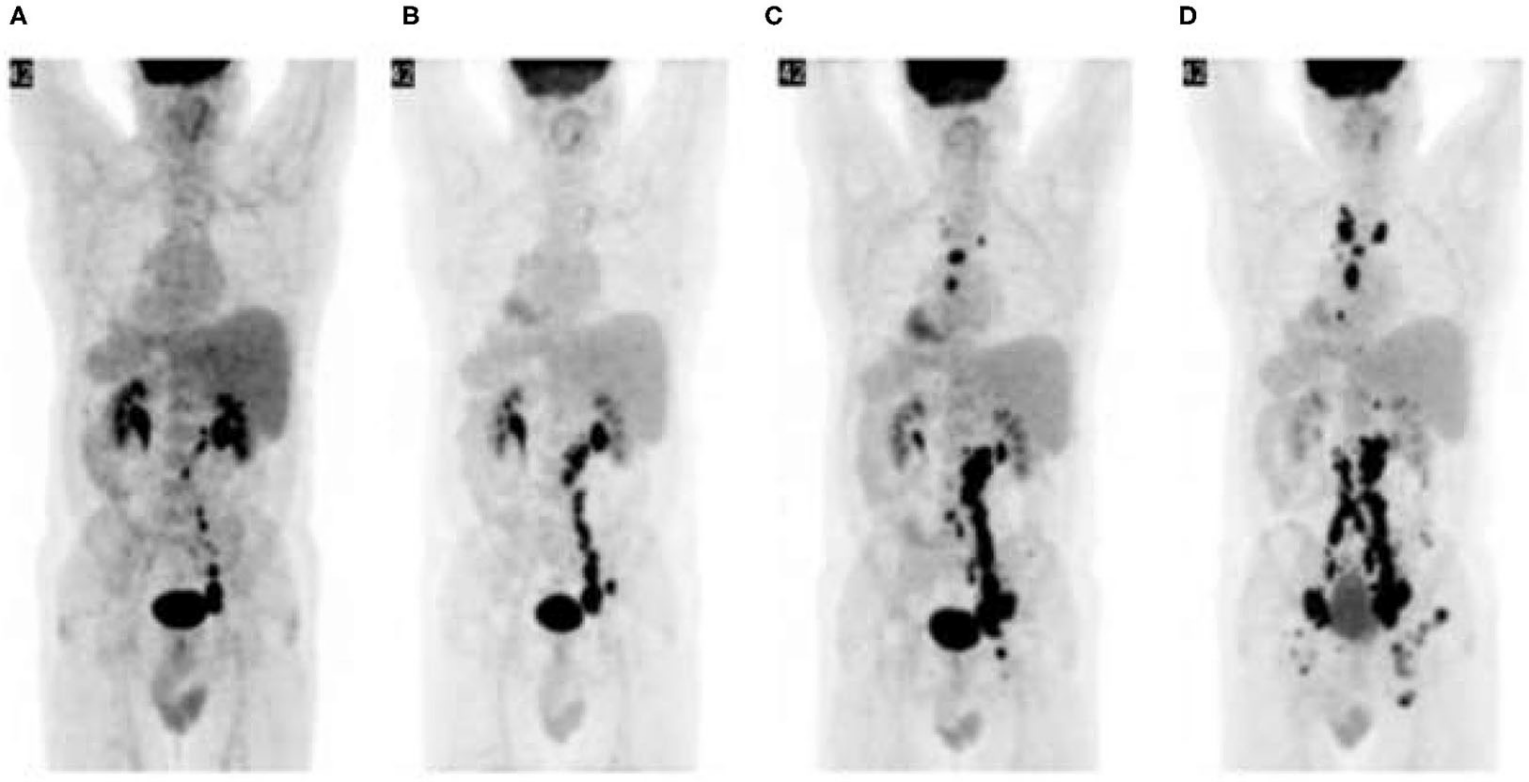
Hyper-progression: Malignant melanoma with failed response to chemotherapy. [18F]FDG PET/CT prior to initiation of ipilimumab shows abdominopelvic lymphadenopathy (A). Repeat PET/CT (B) after two cycles of ipilimumab shows disease progression which is persistent after subsequent treatment cycles (C,D). Immunotherapy was ultimately terminated and other treatment options explored.
